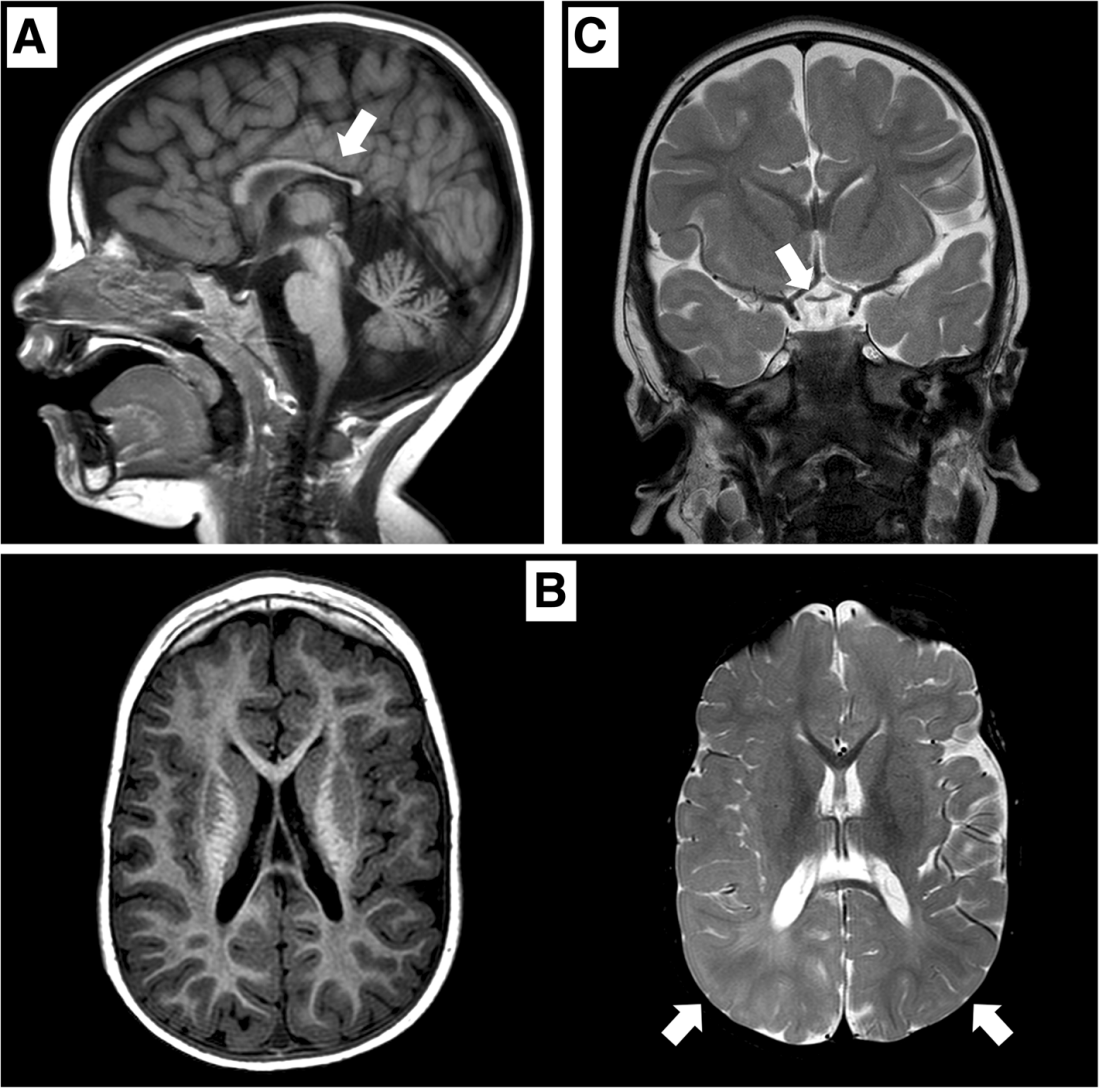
a Sagittal T1-weighted image showing hypoplasia of the corpus callosum (white arrow). b Axial T1-weighted (left) and axial T2-weighted (right) images showing hypoplasia of the corpus callosum and altered, poorly-defined signal in the bilateral subcortical parietal white matter (white arrows). c Coronal T2-weighted image showing hypoplasia of the optic chiasm (white arrow)
